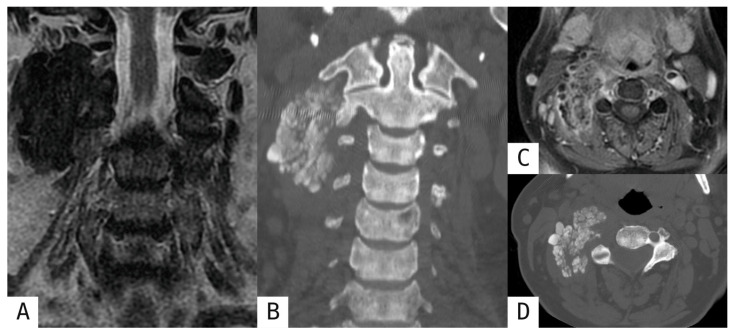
Tumoral calcinosis in the right paravertebral soft tissues. 56-year-old female patient presented with right latero-cervical tenderness. MRI coronal T2-weighted (A), axial T1-weighted FS post-gadolinium (C) and axial and coronal MPR CT reconstructions (B,D) showed a well-defined, lobulated, predominantly homogeneous mass, markedly hypointense in T1-weighted and T2-weighted sequences, consistent with multiple amorphous calcifications (better depicted on CT).
